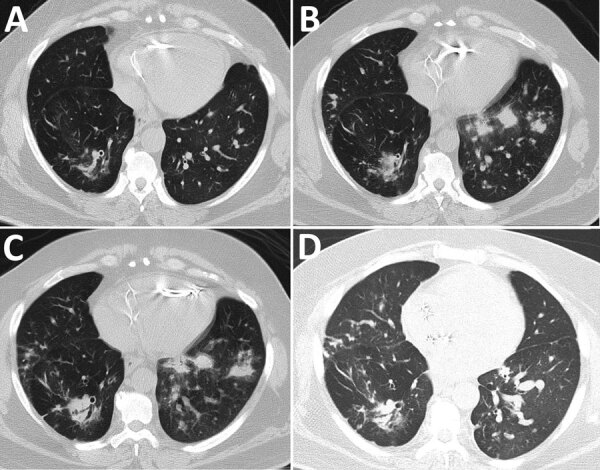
Computed tomography of the chest without contrast showing progression of illness in a 52-year-old immunocompromised man who experienced *Nocardia pseudobrasiliensis* pneumonia after a SARS-CoV-2 infection. A) Scan obtained 4 months before patient sought care. B) Scan on hospitalization day 5 shows development of lung nodules without ground-glass opacities in both lungs. C) Scan on hospitalization day 12 shows progression of nodules on the left lung. D) Scan obtained 28 days post-discharge shows improvement of pulmonary nodules.
